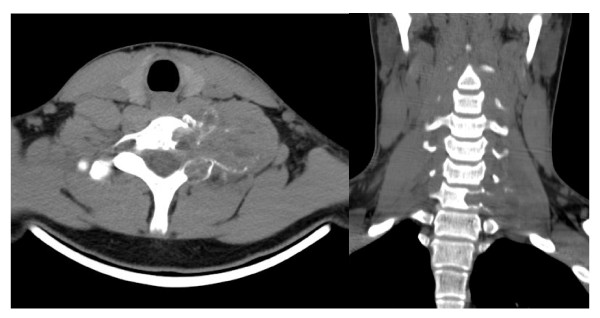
The axial and coronal planes of the CT scan image show the localization and expansion of the bone cyst originating from C7.
